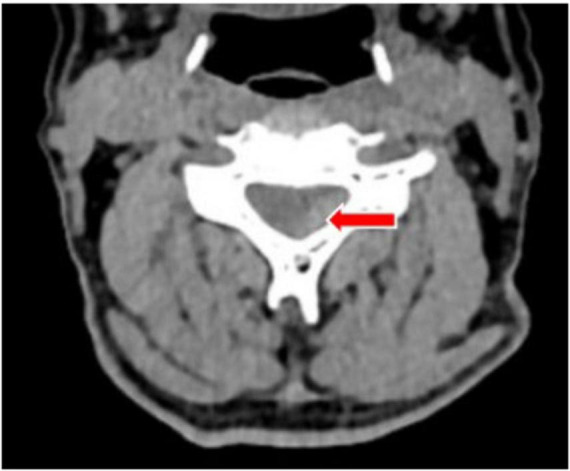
A plain computed tomography (CT) scan showed a strip of high-density shadow in the posterior part of the cervical spinal canal, with a CT value of about 71 HU, and the spinal cord was slightly compressed and displaced in the right front. The site of the lesion is indicated by the red arrow.
